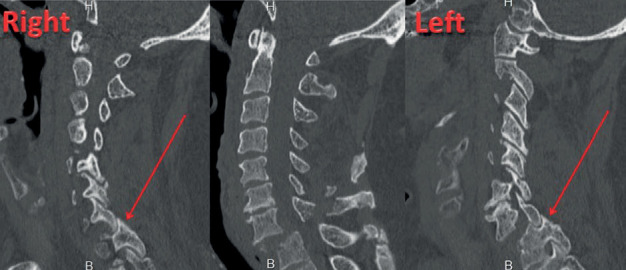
Preoperative CT scan of the patient shows vertebral body fracture of T1 and biarticular dislocation of the cervicothoracic junction.
